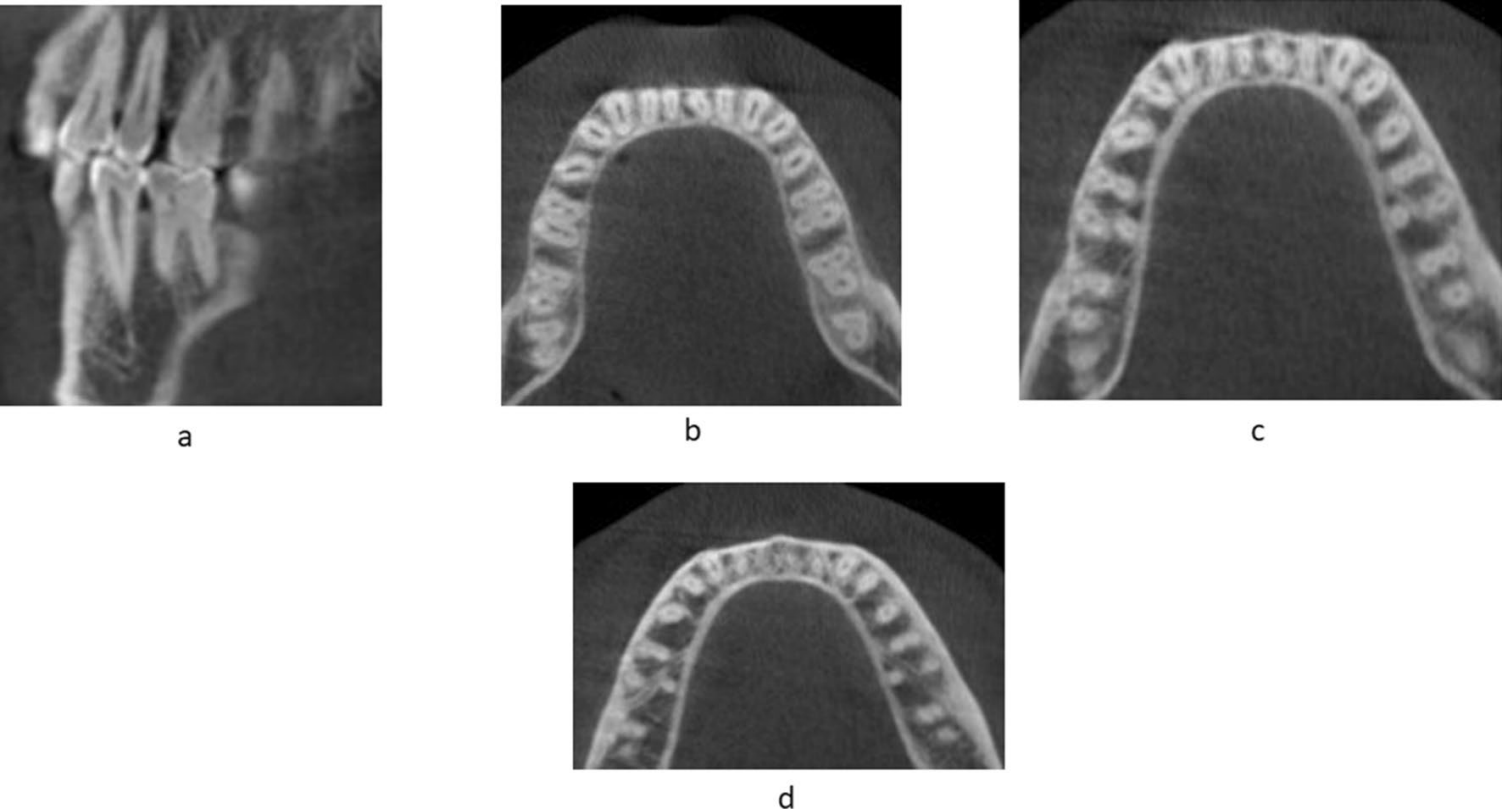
CBCT scans showing DLR in MFMs through various sections from coronal to apical. (a) Sagittal section of mandibular first molar. (b) Coronal third section of the axial view. (c) Middle third section of the axial view. (d) Apical third section of the axial view.
